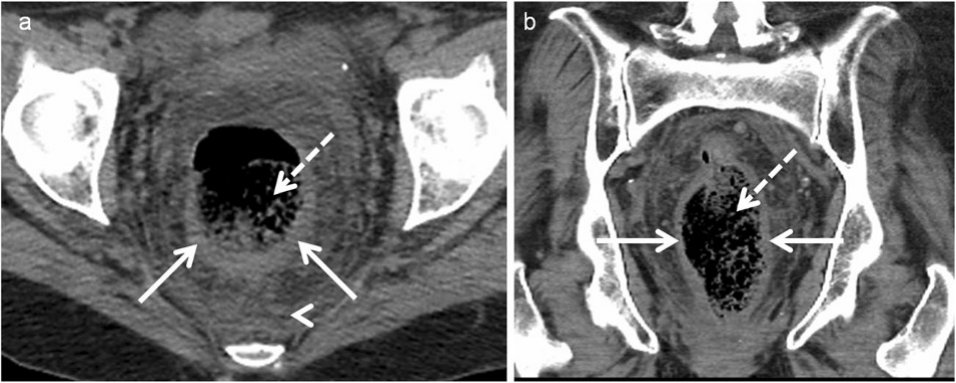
An 81-year-old woman with stercoral colitis. a Axial and b coronal contrast CT reveal impacted faeces (*dashed arrow*) with thickening of the rectal wall along its posterior aspect (*arrows*) with presacral oedema
